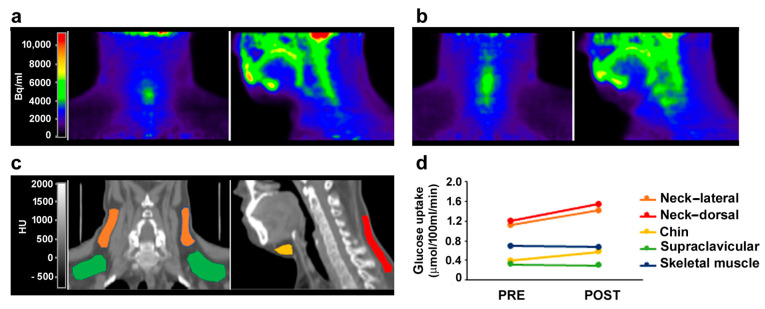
Glucose uptake in a FPLD2 patient undergoing spironolactone treatment. PET-CT images of one FPLD2 patient before (a) and after 6-months of spironolactone treatment (b) are shown. The patient’s neck and head (up to the nose) are visualized in coronal (left panels) and sagittal (right panels) planes in PET (a,b) and co-registered CT (c) images for anatomical reference. PET images show tissue radioactivity, expressed as becquerel per ml of tissue (Bq/mL) (a), whereas CT images show tissue radiodensity expressed in Hounsfield units (HU). Several regions of interest (ROIs) corresponding to fat depots of the neck (lateral and dorsal), chin, and supraclavicular regions were identified in co-registered CT images (c), and ^18^F-FDG extraction rates were measured in PET images. Glucose uptake was calculated as the product of ^18^F-FDG extraction rate and glycemia during the PET scan. Pre- vs. post-treatment levels of cold-induced glucose uptake in these fat depots were compared (d). Glucose uptake in skeletal muscle (trapezius muscle) was also analyzed for comparison as a negative control. The colors in the CT image and in the graph correspond to the anatomical identification of the adipose depots analyzed.
